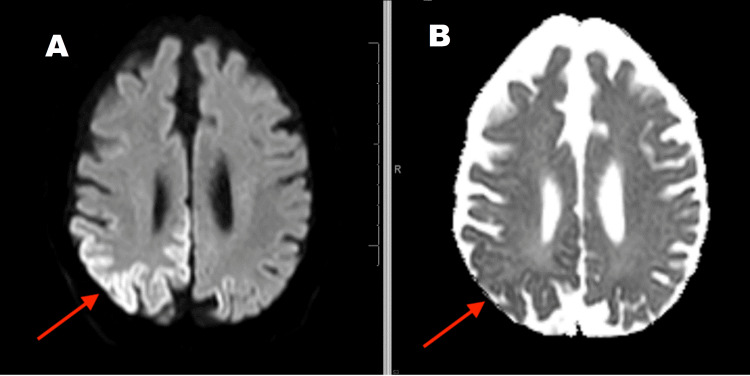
Follow-up MRI Brain after one month shows right superior parietal region "cortical ribboning" in the DWI image (A) and persistent ADC changes (B) (A) Diffusion-weighted imaging (DWI) sequence; (B) Apparent diffusion coefficient (ADC) sequence
